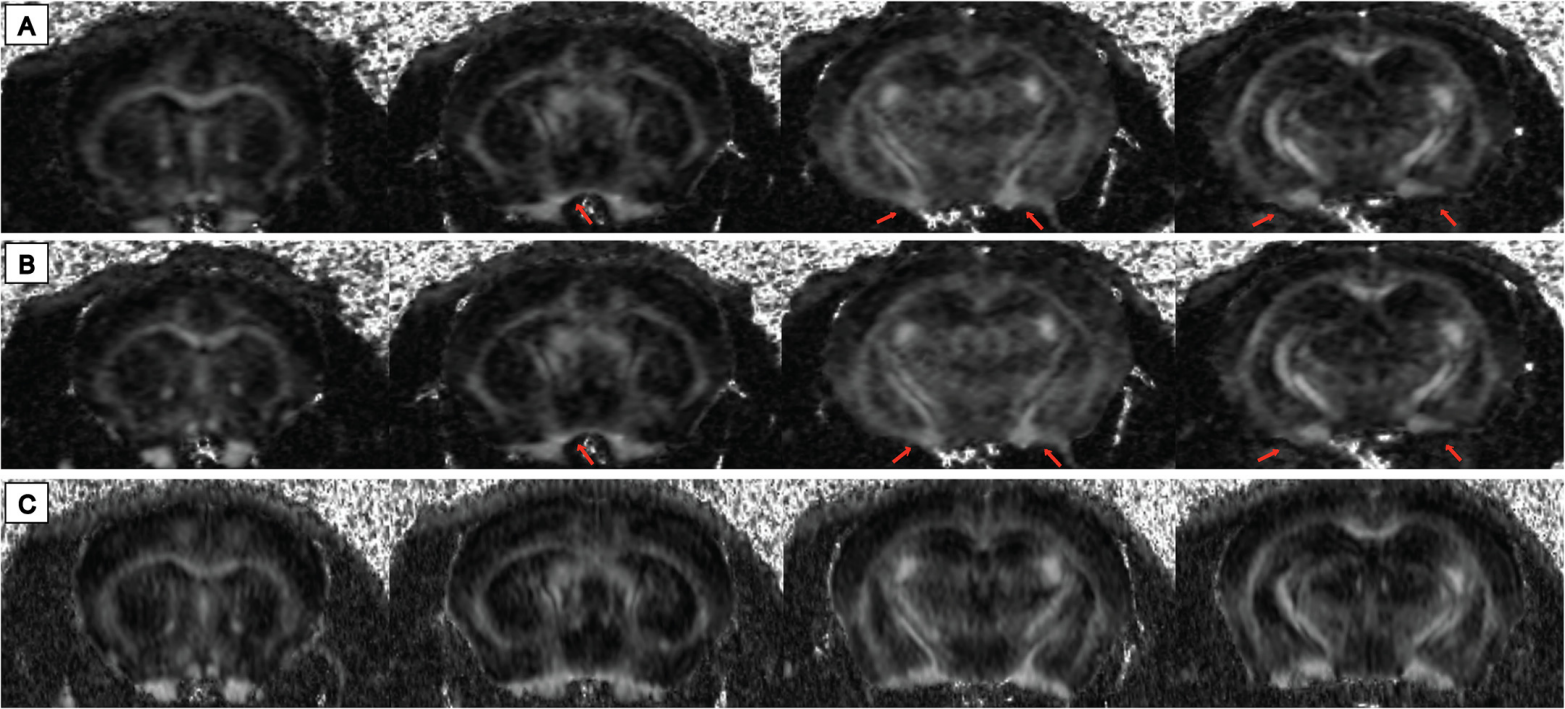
FA map comparison between *in vivo* and in situ segmented EPI-DWI, and *in situ* SE-DWI. Rostral to caudal brain slices of FA maps reconstructed form *in vivo* segmented EPI-DWI (A), *in situ* segmented-EPI DWI (B) and *in situ* SE-DWI (C) acquired at 0.6 mm slice thickness. Distortion artefacts observed in *in vivo* and *in situ* segmented EPI-DWI are shown with red arrows.
